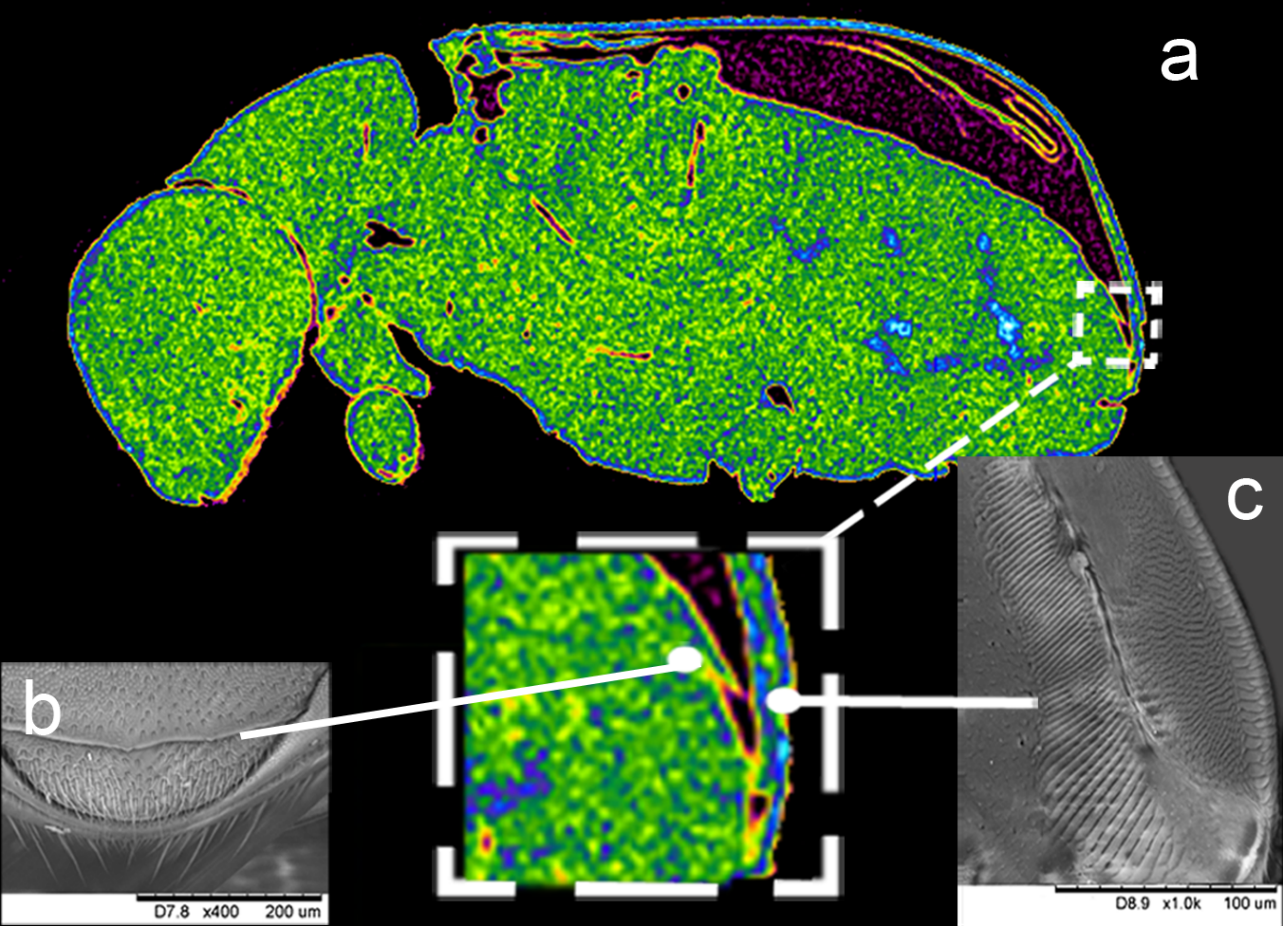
General view of elytro-tergal stridulatory apparatus morphology. (A) Longitudinal section of the imago prepared using an X-ray microtomography device; image of the elytron-tergite stridulatory apparatus of males; (B) detailed structure of the plectrum; (C) pars stridens structure.
